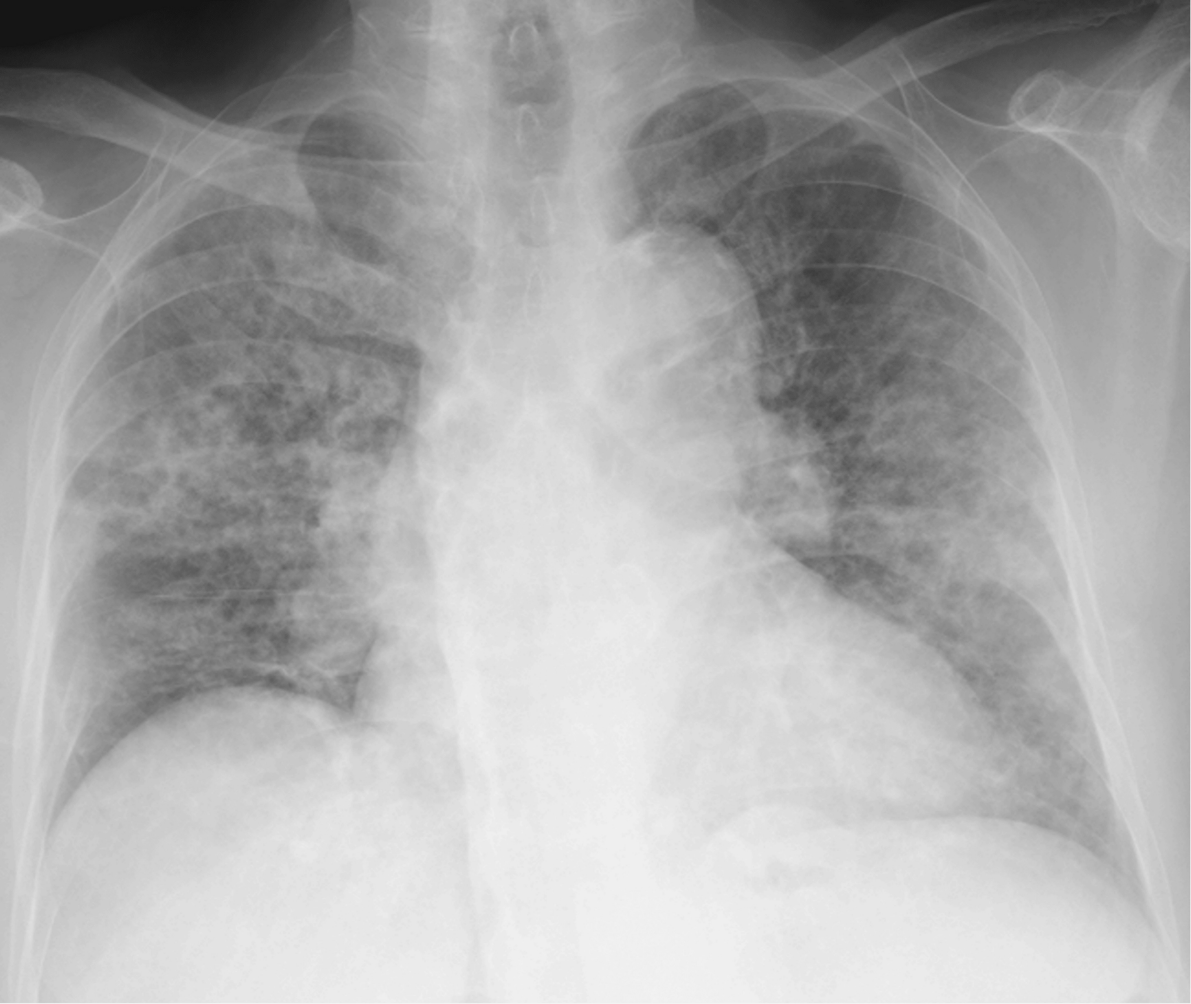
Chest X-ray at the initial visit Chest X-ray showed bilateral ground-glass opacities.
